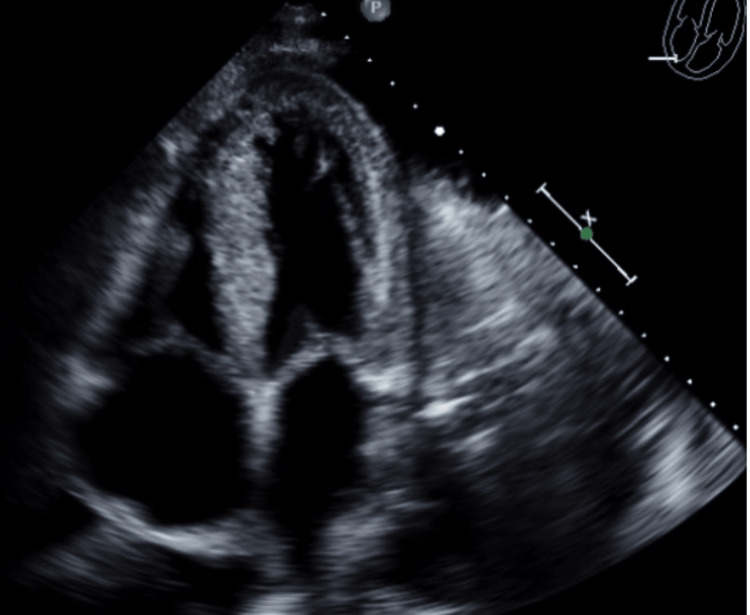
Transthoracic echocardiogram (2D ECHO) done significant for moderate-to-severe bi-ventricular hypertrophy, and systolic function (LVEF >65%), indeterminant diastolic function due to underlying atrial fibrillation LVEF: Left ventricular ejection fraction
